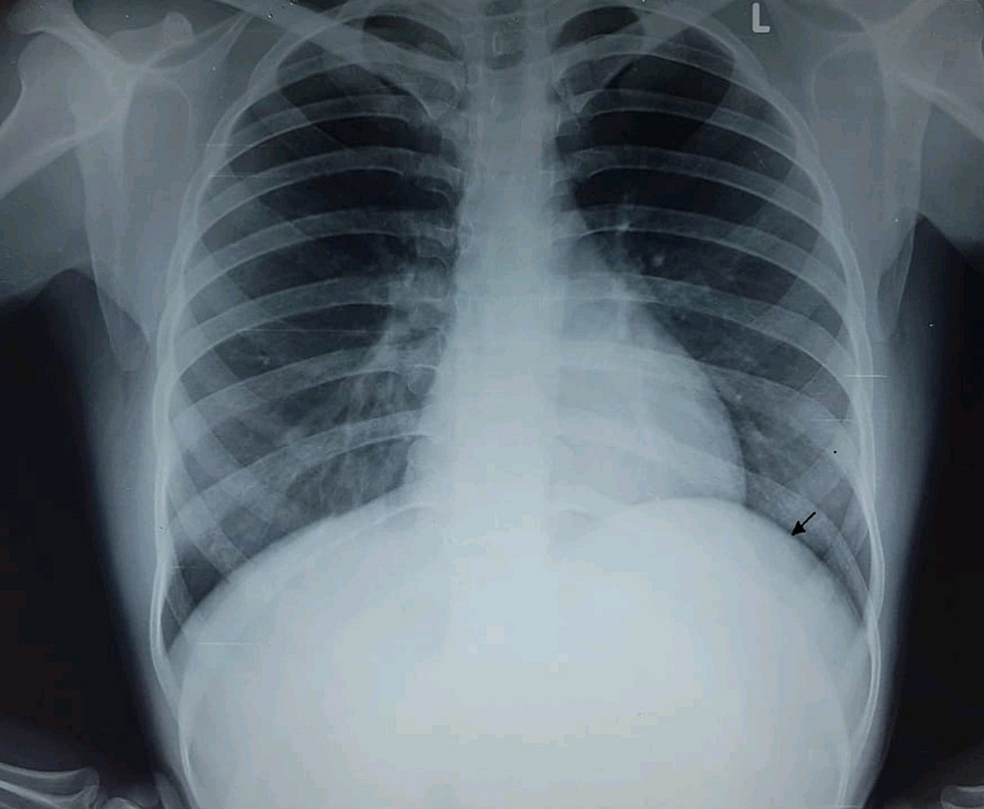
X-ray chest (PA view) depicting raised left hemidiaphragm due to a large cystic lesion left hypochondrium (arrow) PA: posteroanterior
